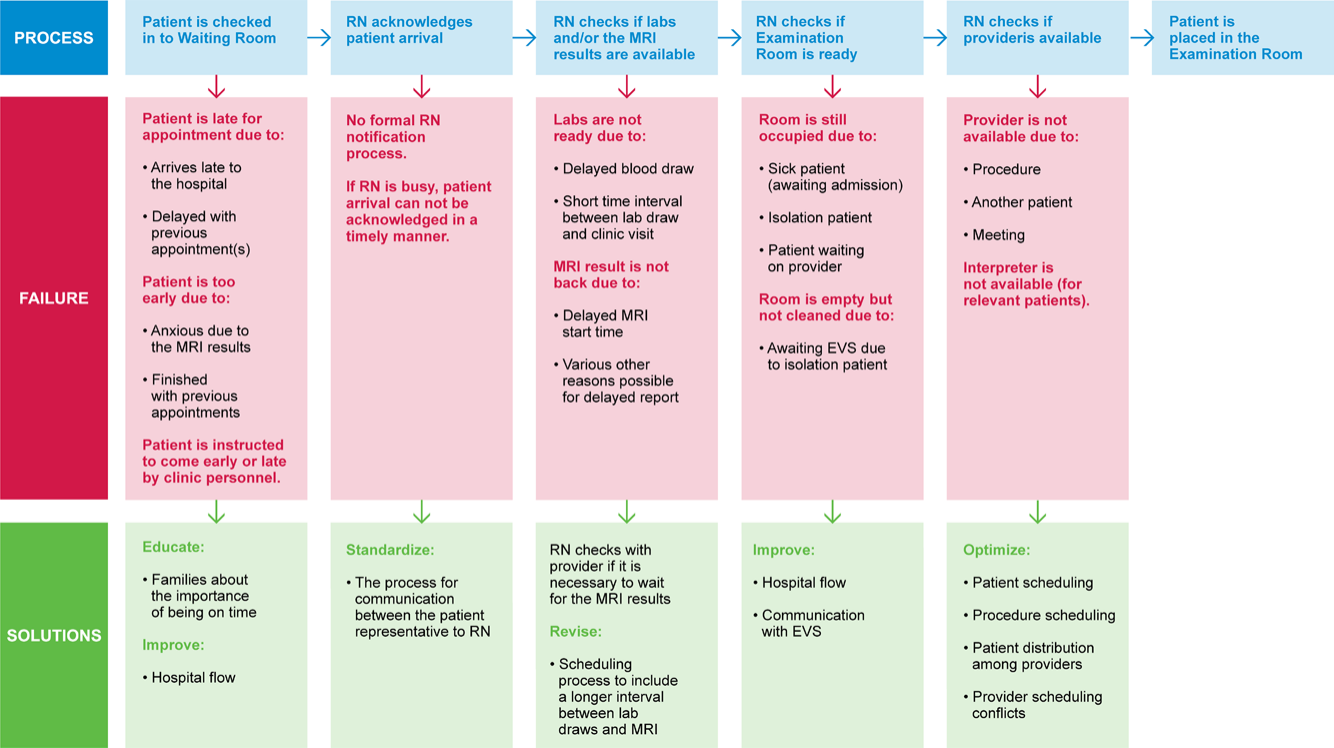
Process flow for patient arrival to the examination room in the NO clinic. EVS, environmental services; Labs, laboratory results; MRI, magnetic resonance imaging; RN, registered nurse.
